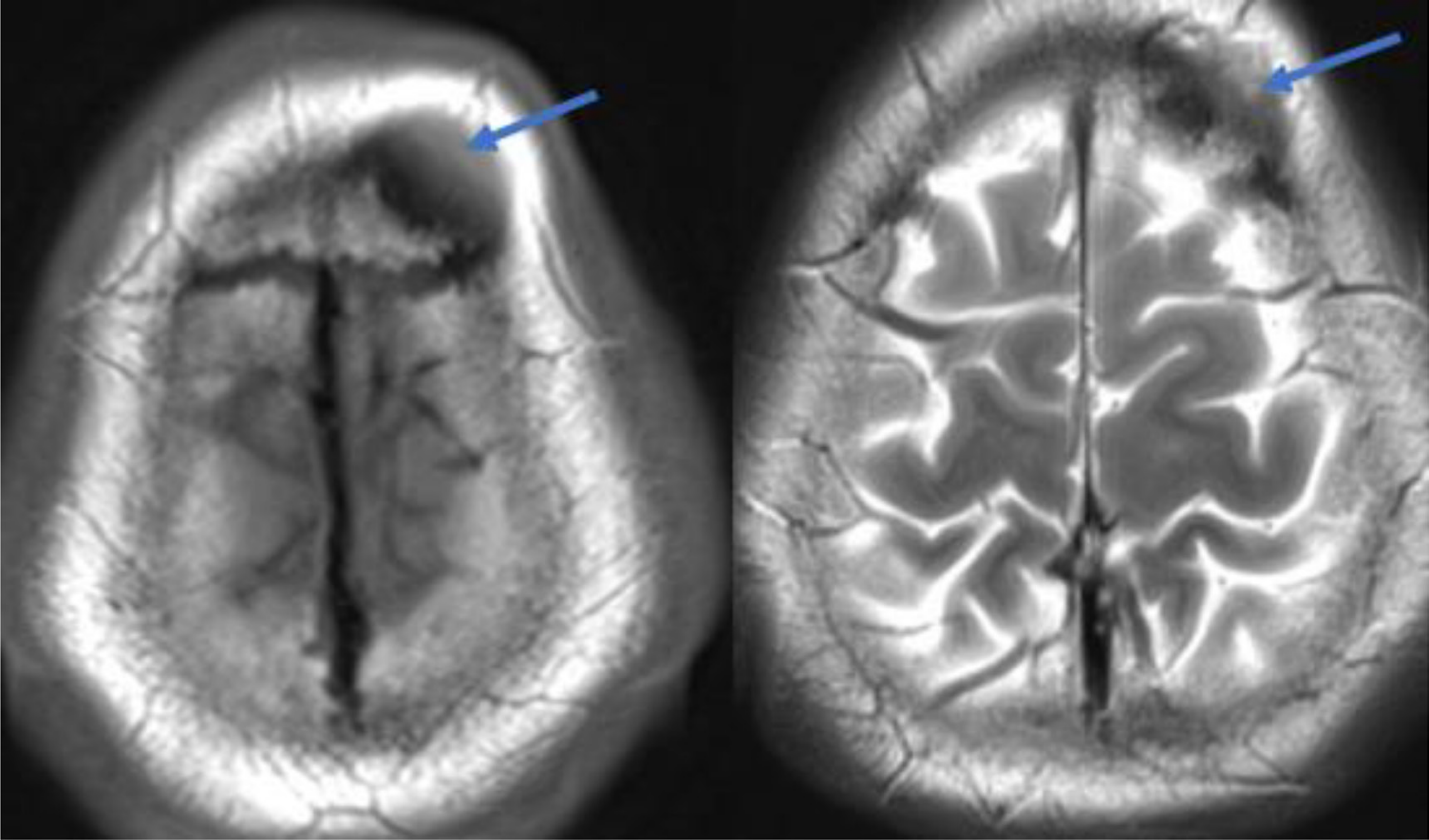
MRI T1WI (the left image) and T2WI (the right image) show the subcutaneous lesion, which is hypointense in both sequences (blow arrows).
